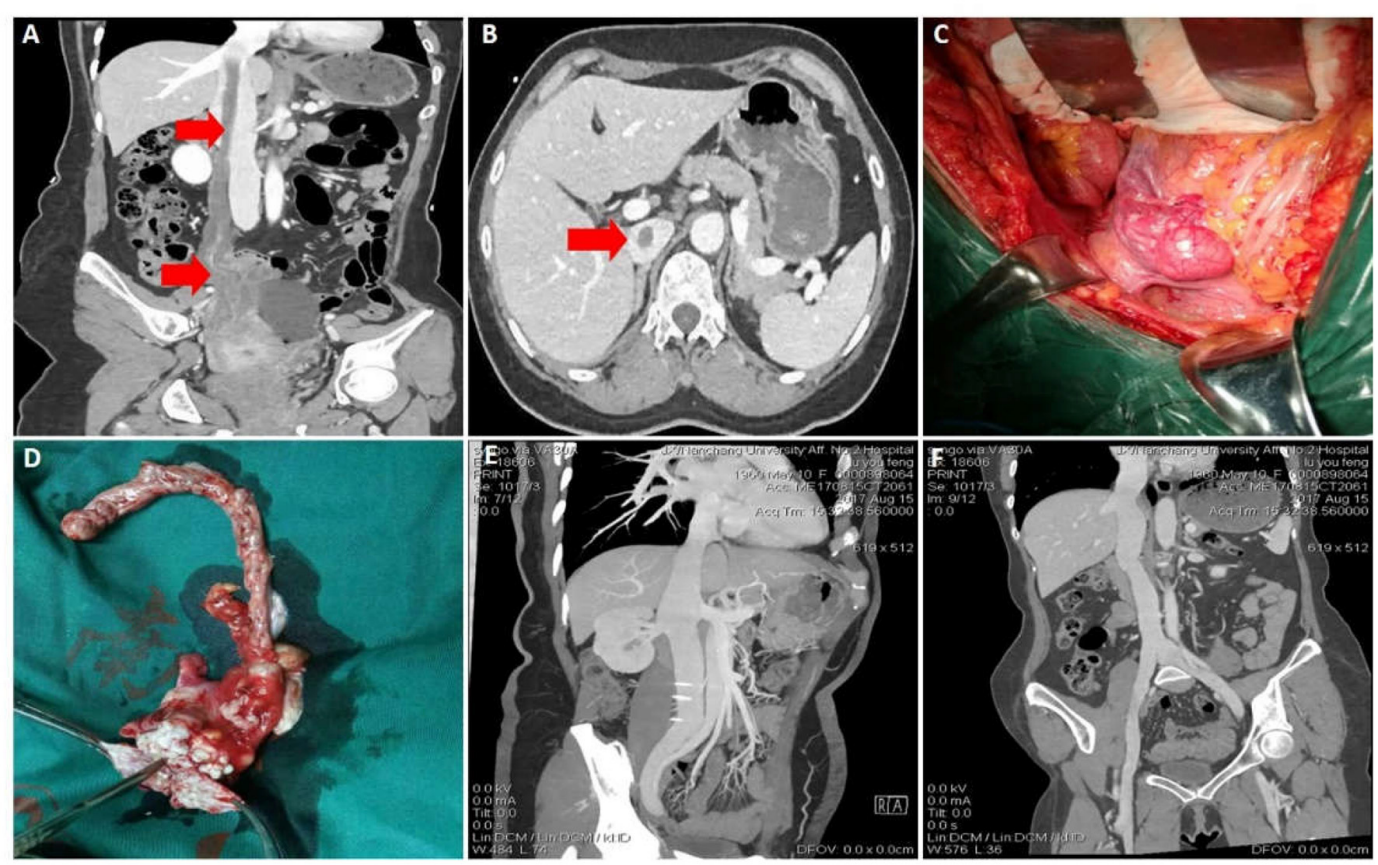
Data of case 2 before, during, and during follow-up. (A,B) Preoperative CTV and abdominal CT scan showed a mass extended through the right ovarian vein to the huge occupying lesion in the inferior vena cava. (C) Inferior vena cava tumor and right ovarian vein were removed, and the right adnexa and part of uterus were also removed. (D) The intact mass was removed. (E,F) Eight months after surgery, CTV confirmed that the inferior vena cava was unobstructed and the prognosis was good.
